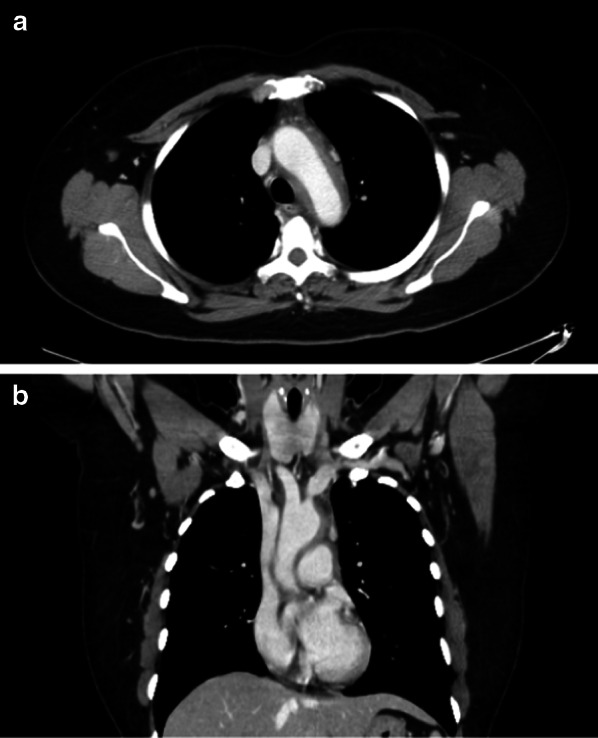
Computed tomography (CT) scan on admission. CT scan on day 11 reveals diffused wall thickening centering on the aortic arch. Vasculitis was suspected of causing the fever. a Axial plane; b coronal plane
